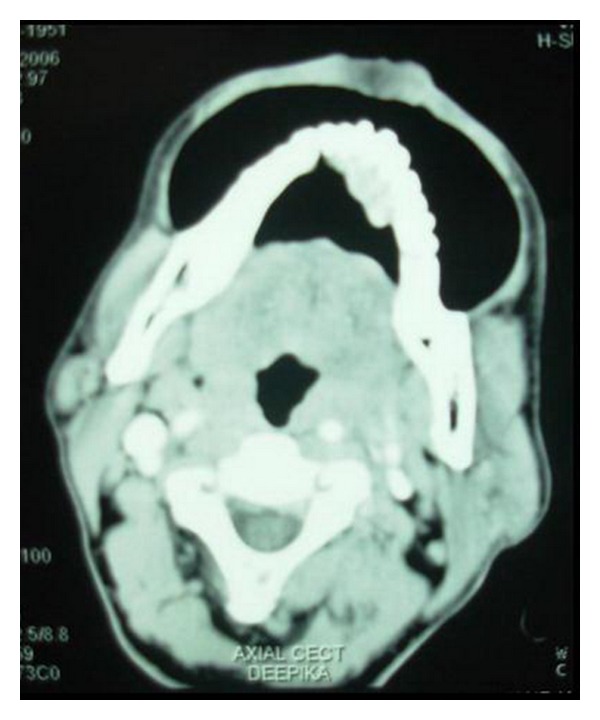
Blow mouth axial CT scan showing enhanced growth.
